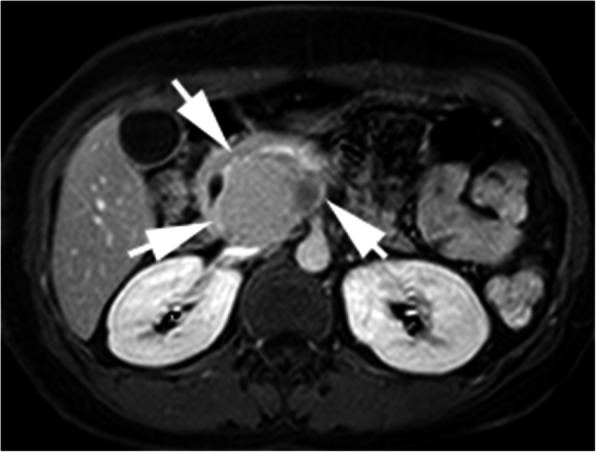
The cystic wall and thin septa was showed with moderate degree of enhancement on delayed phase (arrow), dynamic contrast enhancement pattern of lesion was the same as CT enhancement
